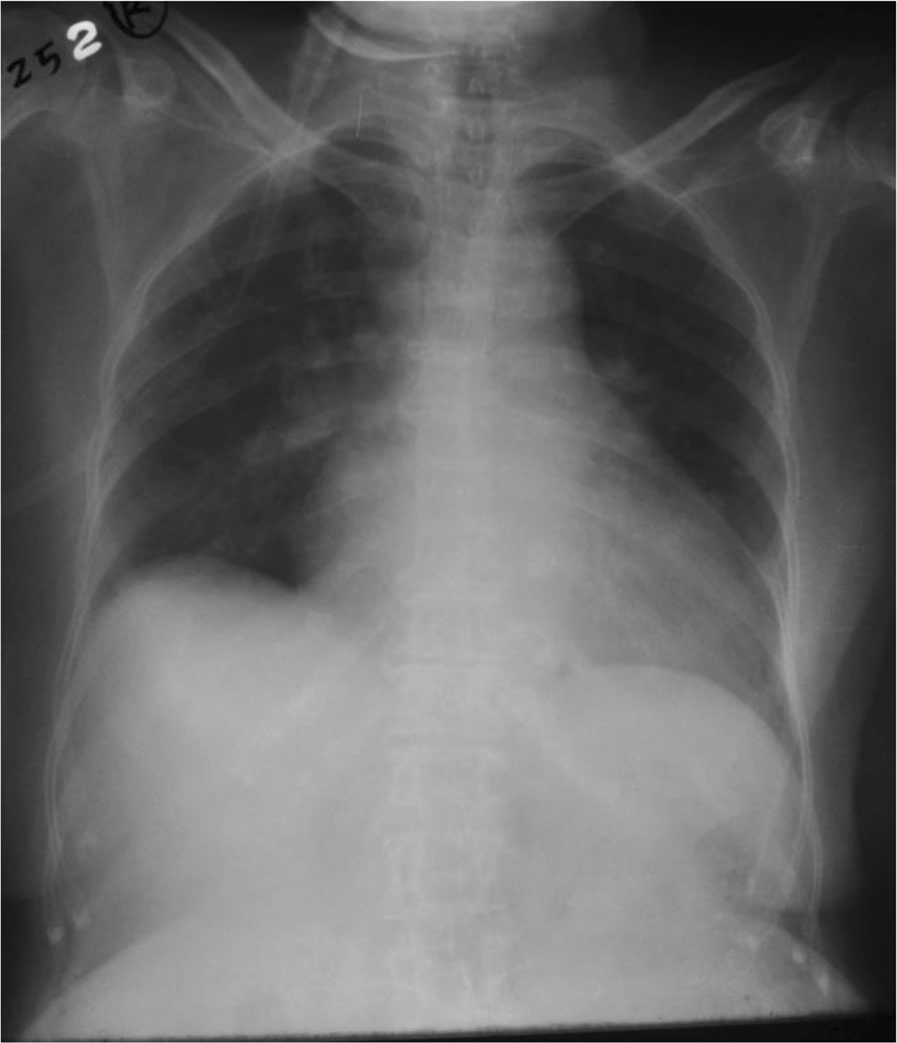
Chest X-ray taken after 12 hours. This X-ray was taken after intravenous furosemide/glyceryl trinitrate infusion
